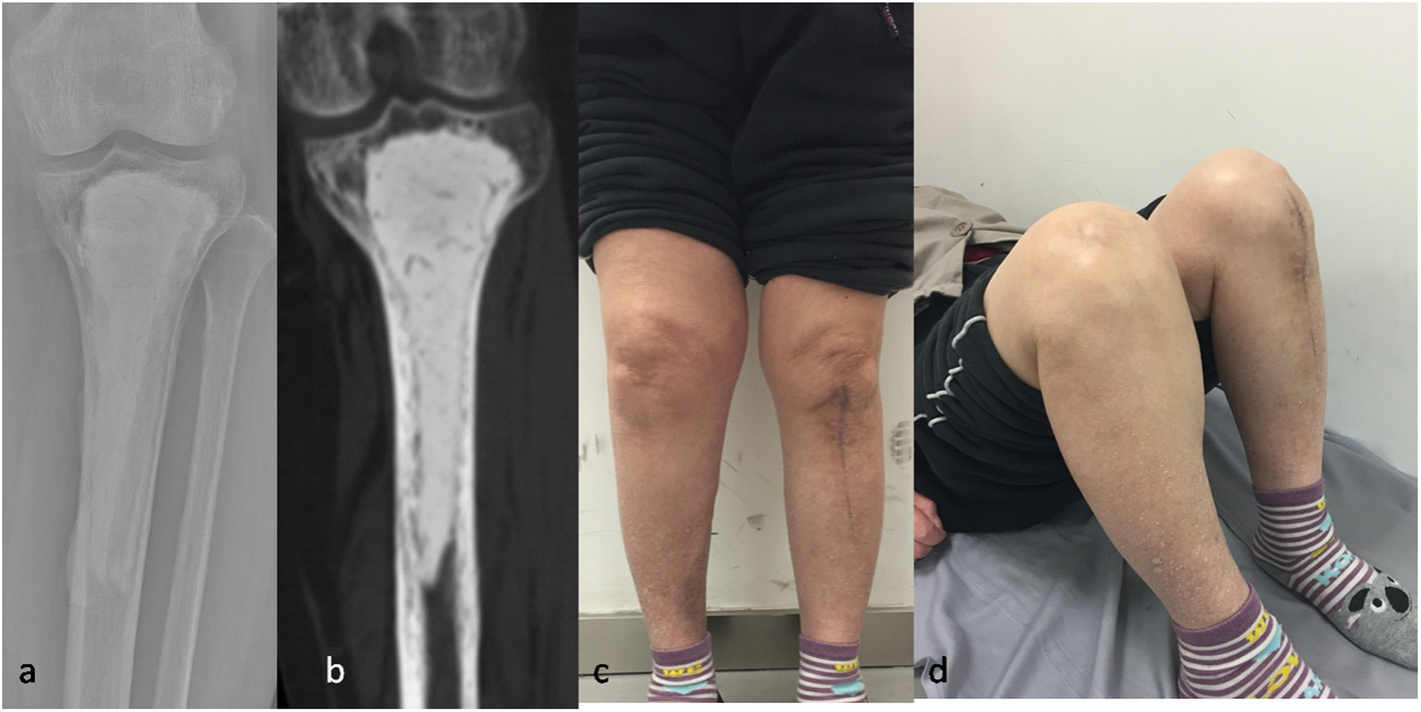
a-b At the 3-year follow-up, no excessive bone loss was observed on X-ray film and CT scan. c The patient achieved complete weight-bearing ability without pain; no sign of recurrence of the infection was observed. d The patient exhibited normal range of motion of the affected knee
